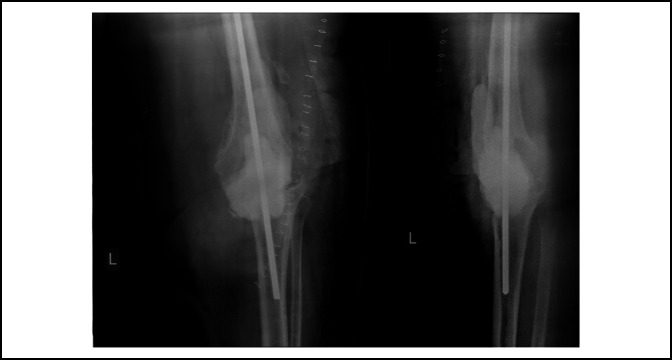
Anteroposterior and lateral radiographs of a knee before arthrodesis.
